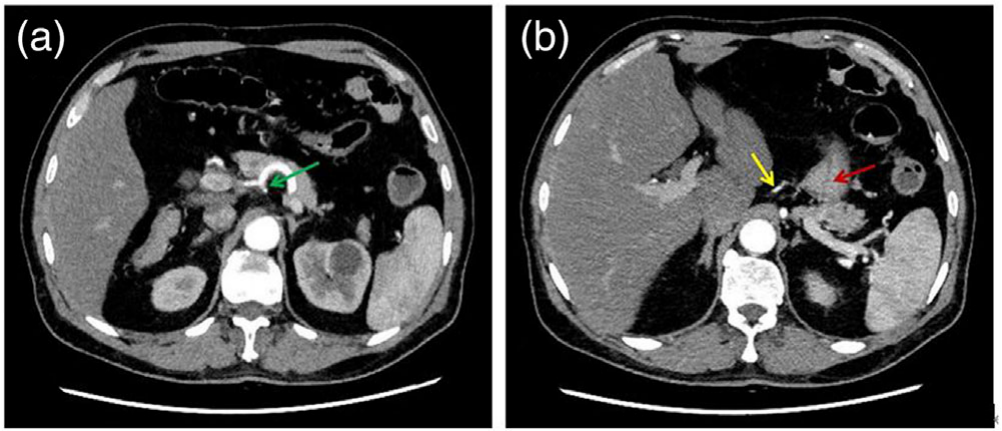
Abdominal enhanced computed tomography scan. (a) The left gastric artery from the celiac trunk (arrow). (b) The nutrient artery of the tumor from the left gastric artery (yellow arrow) and the residual stomach (red arrow).
